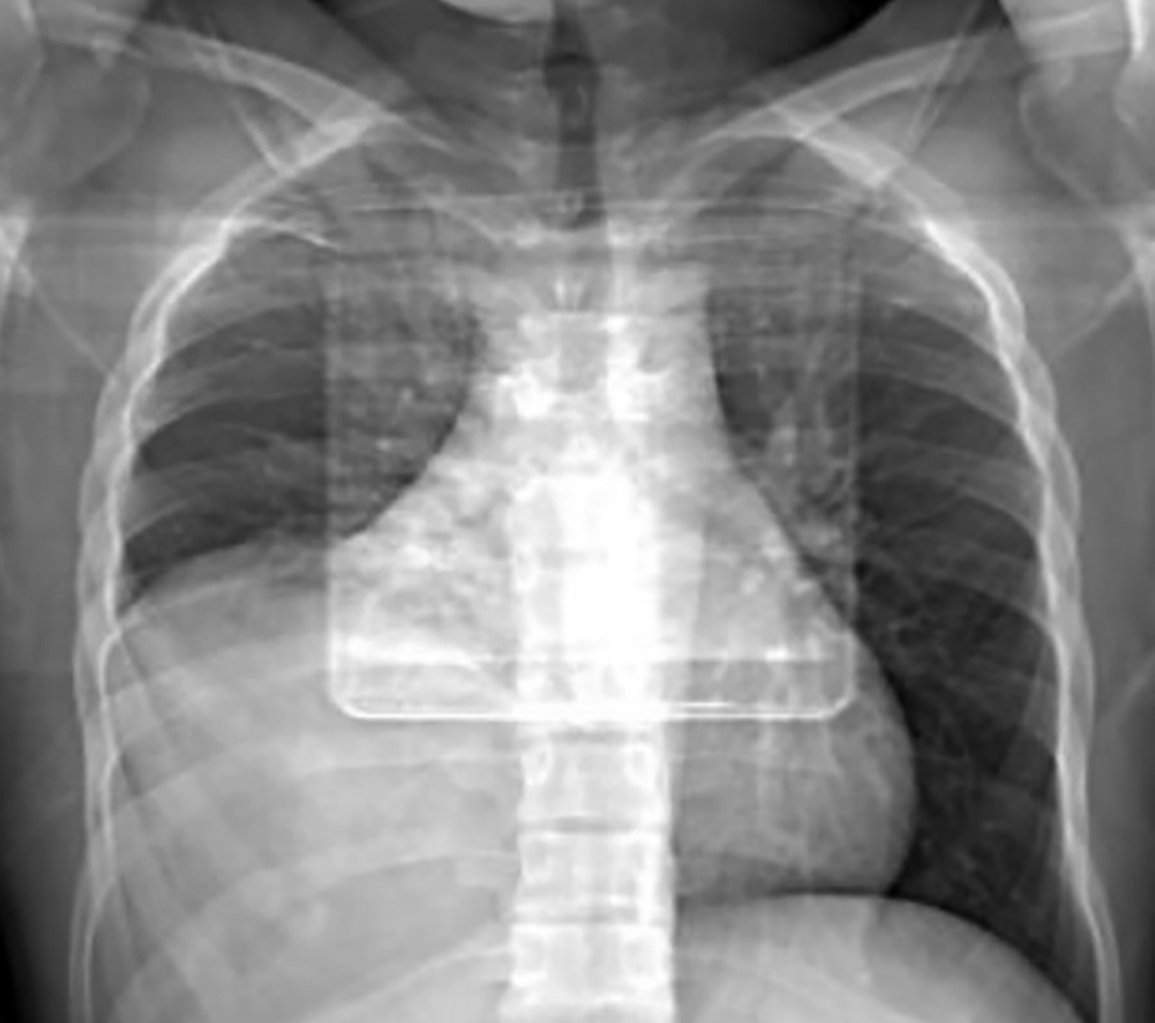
Chest CT scout view shows an opacity in the right lower hemithorax with minimal shifting of the heart towards the left side.
